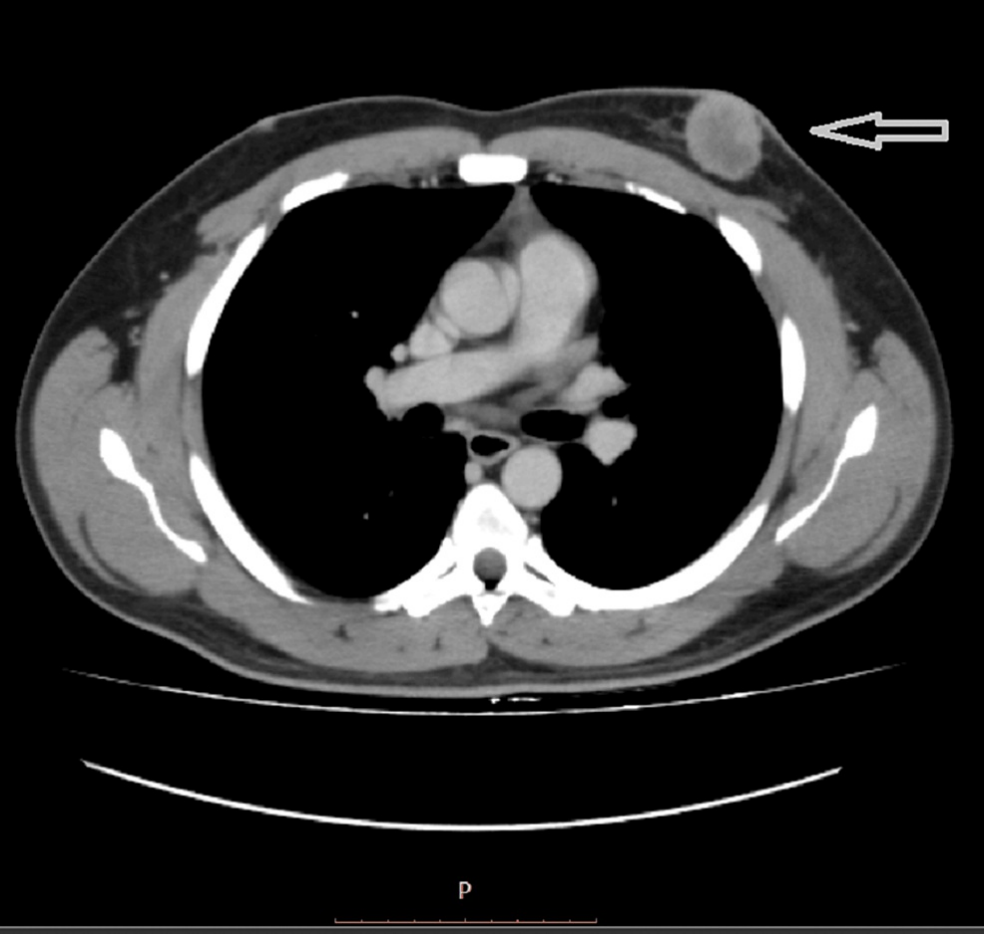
Computed tomography for the metastatic workup The left breast demonstrated a large, enhancing circumscribed retroareolar mass measuring 3.4 cm in maximum dimension associated with ipsilateral metastatic axillary node, with the largest having a short axis diameter of 2.5 cm. There was a plump benign-appearing subcarinal lymph node with a short axis diameter of 1.1 cm along with a right hilar subcentimeter calcified node. The visualized lower cervical and the right axilla and supraclavicular recesses were clear. There were no suspicious pulmonary or pleural focal abnormality or effusions. There was no evidence of hepatopulmonary or osseous metastasis.
